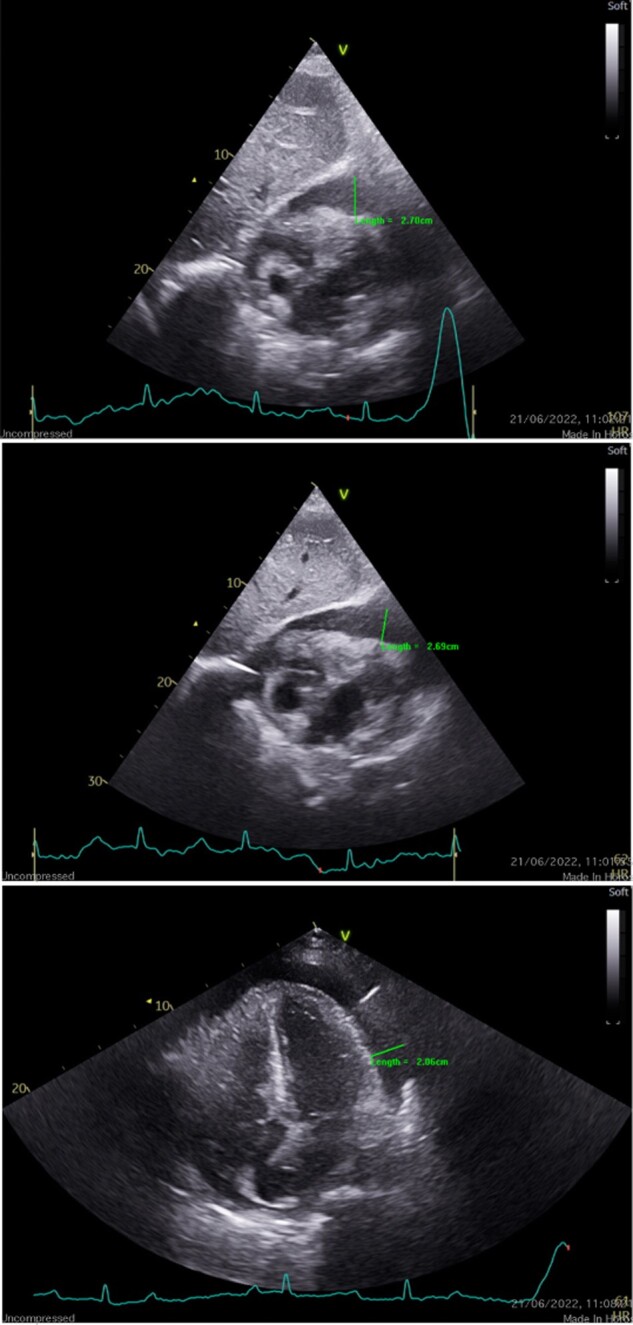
Transthoracic echo shows a large circumferential pericardial effusion measuring 2.7 cm at its largest, with evidence of right chamber collapse.
